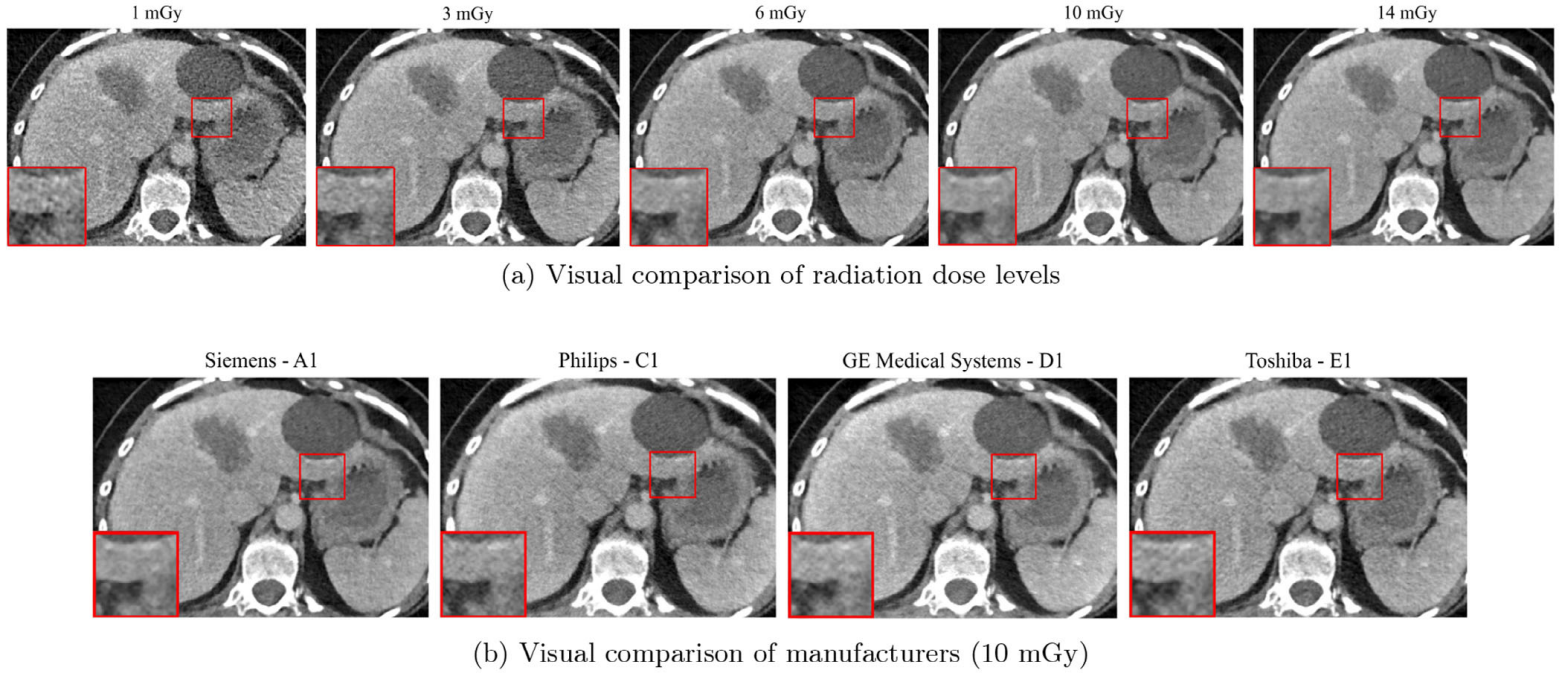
Axial CT slices acquired under two experimental settings. (a) Same scanner (Siemens SOMATOM definition Edge ‐ A1) with IR reconstruction at different radiation dose levels (1, 3, 6, 10, and 14 mGy). (b) Same radiation dose (10 mGy) and IR reconstruction, but across four different manufacturers (Siemens, Philips, GE Medical Systems, and Toshiba). A zoomed‐in patch is shown in each image to highlight texture differences for qualitative comparison (level=50, window=400).
